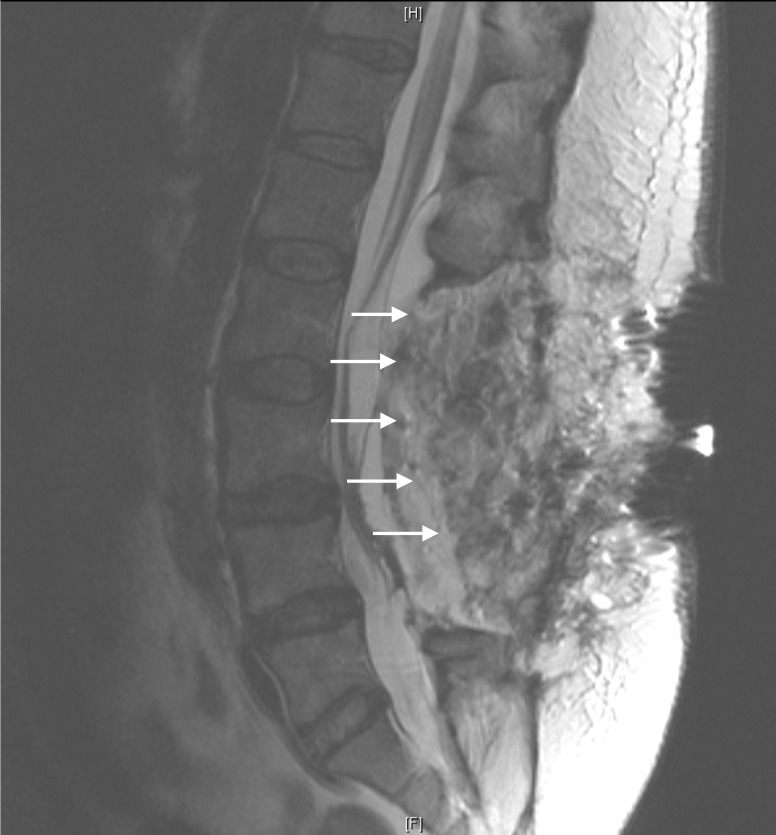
Post-Operative Sagittal MRI of Lumbar Spine
